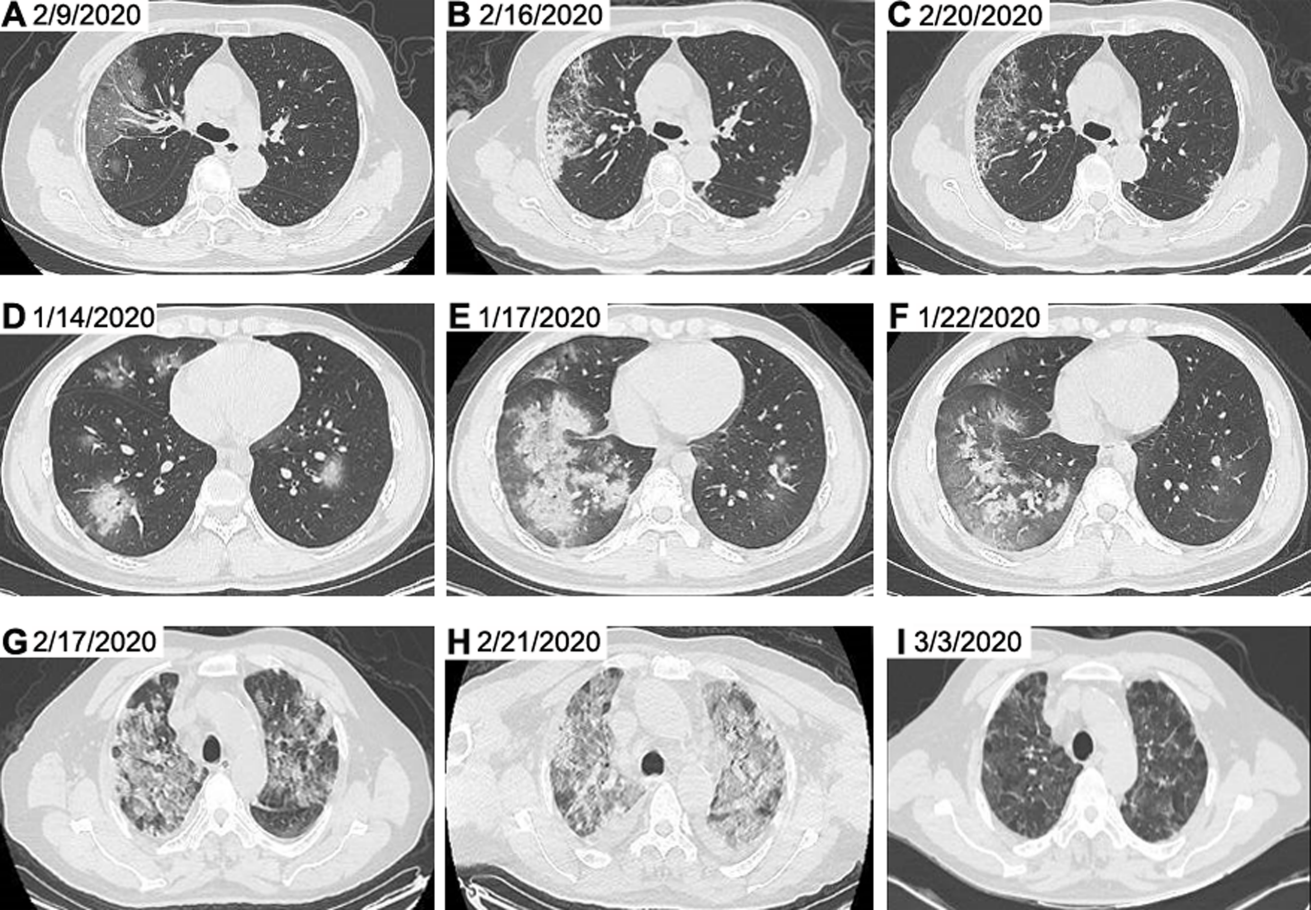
Representative chest CT images of patients with COVID-19. Chest CT images from a 65-year-old woman with non-severe disease on day 9 after symptom onset (A), on day 16 after symptom onset (B) and on day 20 after symptom onset (C) were shown. Chest CT images from a 23-year-old man with severe disease on day 7 after symptom onset (D), on day 10 after symptom onset (E) and on day 15 after symptom onset (F) were presented. Chest CT images from a 62-year-old man with critically severe disease on day 13 after symptom onset (G), on day 17 after symptom onset (H) and on day 28 after symptom onset (I) were shown. The chest CT images indicated multi-focal ground glass opacity and parenchyma consolidation, mainly involving subpleural regions of both lungs in patients with COVID-19. CT, computed tomography.
